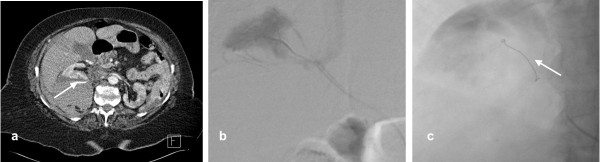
a) A 76 year old lady on warfarin presented with abdominal and back pain following a fall. Contrast enhanced axial CT demonstrates retroperitoneal haematoma associated with a ruptured right kidney and evidence of active contrast extravasaion (arrow). b) Selective catheterisation of the right kidney showed a bleeding focus in the upper pole. c) The branch to the upper pole was selectively catheterised and embolised using a single platinum coil (arrow). Post procedure renal arteriogram demonstrated cessation of haemorrhage.
